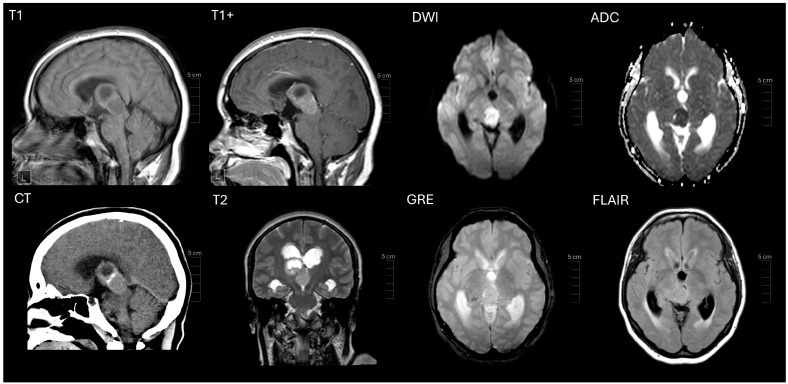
Initial imaging demonstrating an enhancing (T1 and T1+), diffusion-restricting (DWI and ADC), partially calcified (CT), partially cystic (CT), T2-intermediate (T2), tectal-intraventricular mass causing obstructive hydrocephalus (GRE and FLAIR).
